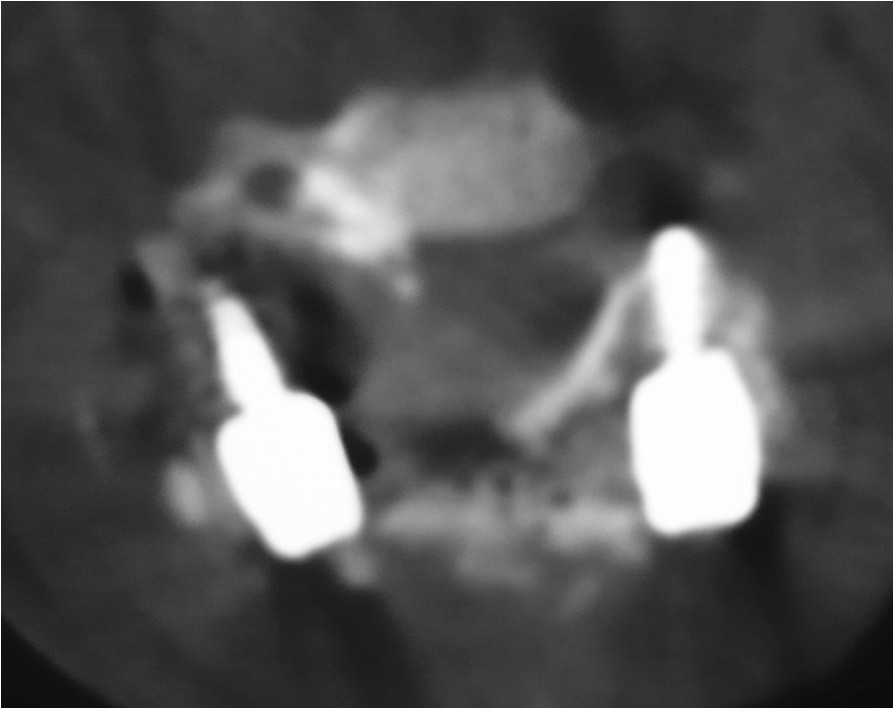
At 1-year follow-up, the CT scan shows no evidence of tumor recurrence.
